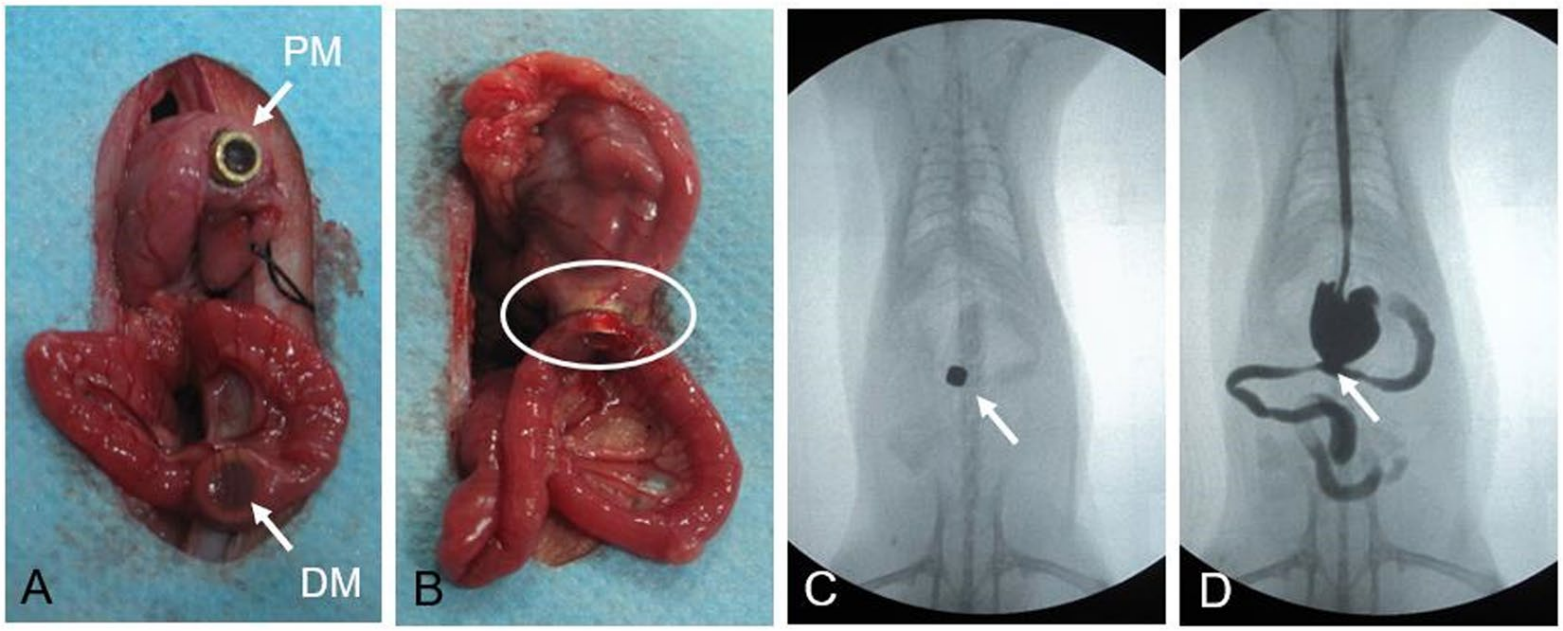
(A) The parent magnetic ring and the daughter magnetic ring; (B) The parent and daughter magnetic ring are attracted to one another; (C) Abdominal fluoroscopy shows the position of the magnetic rings; (D) Upper gastrointestinal contrast radiography showed anastomotic patency.
